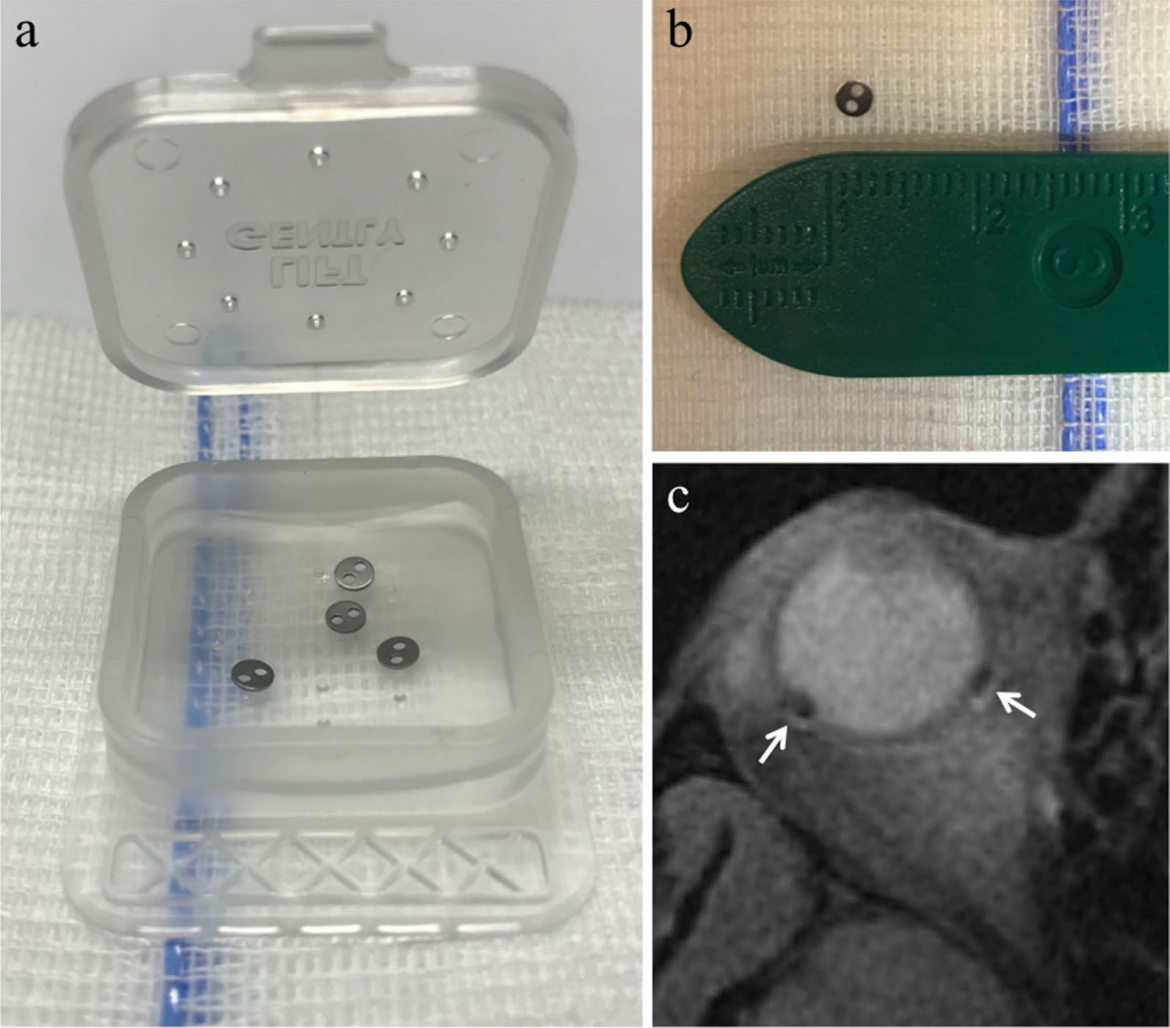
Tantalum clips for proton-beam radiotherapy. a, b Tantalum clips (2.5 mm in diameter). c Fat-suppressed T1-weighted image shows the artefact produced by the clips (white arrows); the artefact is negligible since tantalum is a nonmagnetic metal
